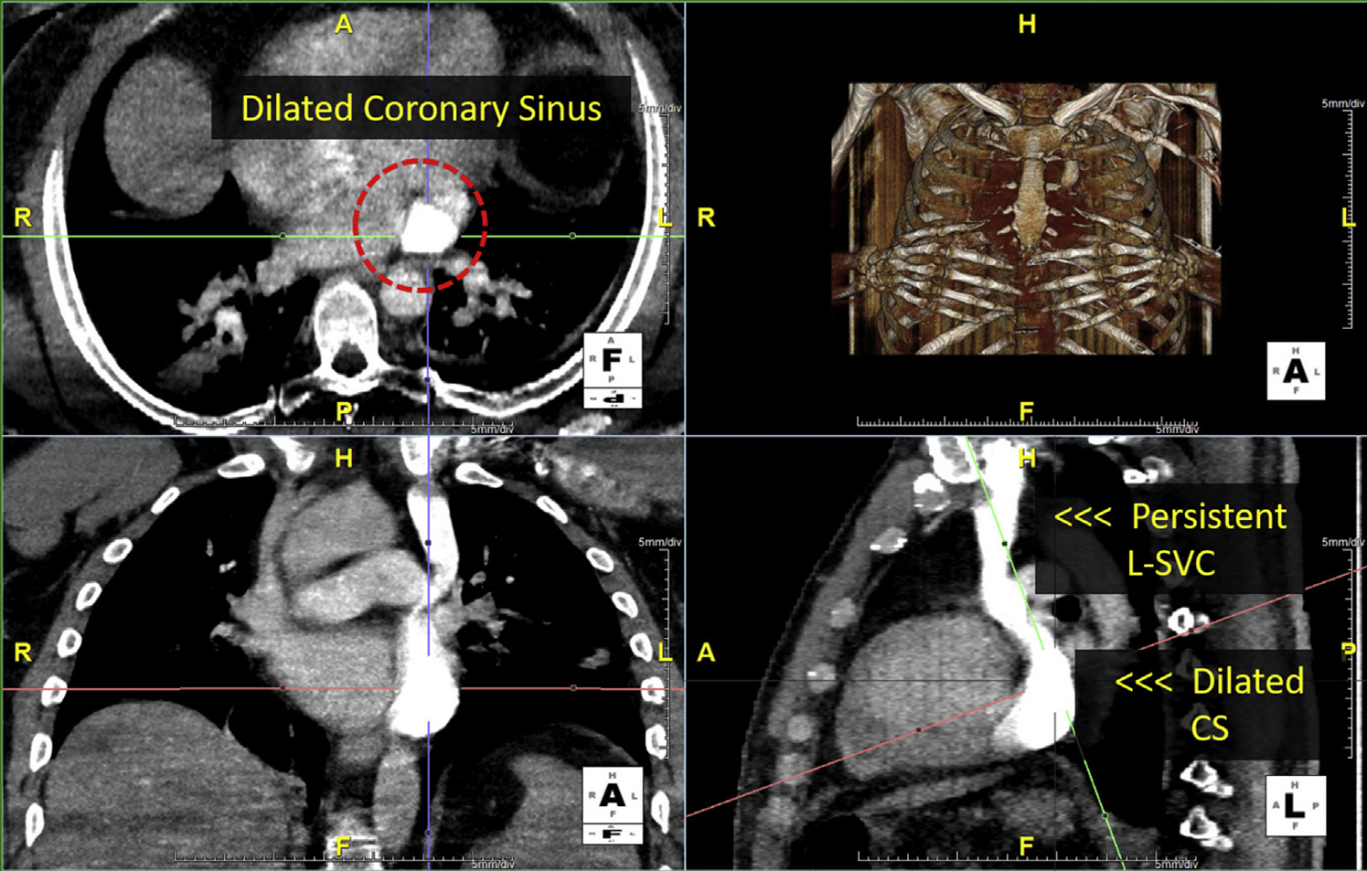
Case 5: R-SVC draining into the LA. Cardiac CT with axial, coronal, and sagittal views. Subsequent CCT imaging with contrast identified a persistent L-SVC draining into a notably dilated CS. The dilated CS is located at the intersection of cross hairs. The identification of an L-SVC on CCT imaging confirms the suspected diagnosis of an L-SVC based on the dilated CS seen on TEE. *CS*, Coronary sinus.
